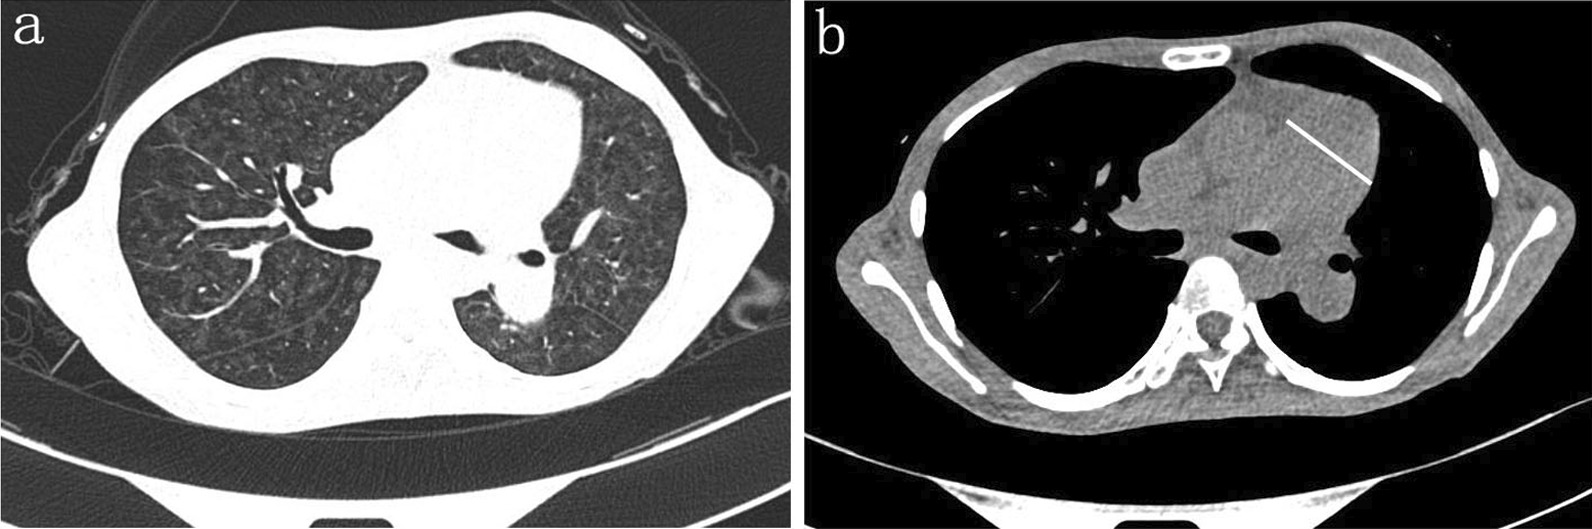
Representative chest high-resolution CT image revealing ground-glass opacities and a dilated pulmonary main trunk in a patient who has PVOD concurrent with Sjogren syndrome. The white line across the main pulmonary artery shows the dilated main pulmonary artery, with an estimated inner diameter of 38 mm
